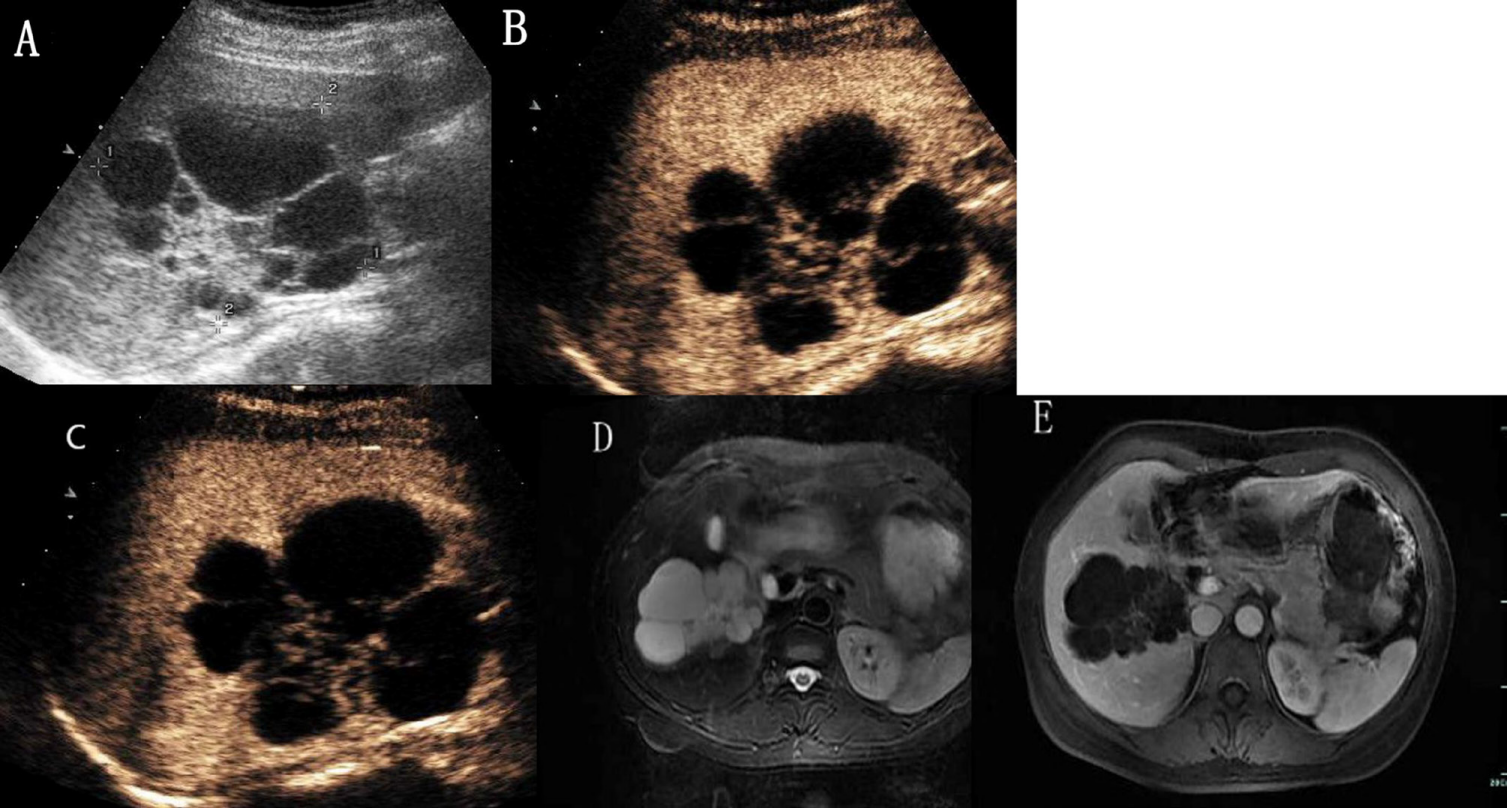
Hepatic hemangioma with septa enhancement in all vascular phases (A) Ultrasound revealed a mix-echoic mass comprised of a multilocular cystic part in the periphery and a stellate echogenic part in the centre. (B) and (C) CEUS showed isoenhanced with septa and stellate part, no enhancement with cystic part. (D) The lesion showed bright signal intensity on T2-weighted MR images. (E) On enhanced magnetic resonance, the septa part enhanced with contrast and the periphery cystic part was not enhanced.
